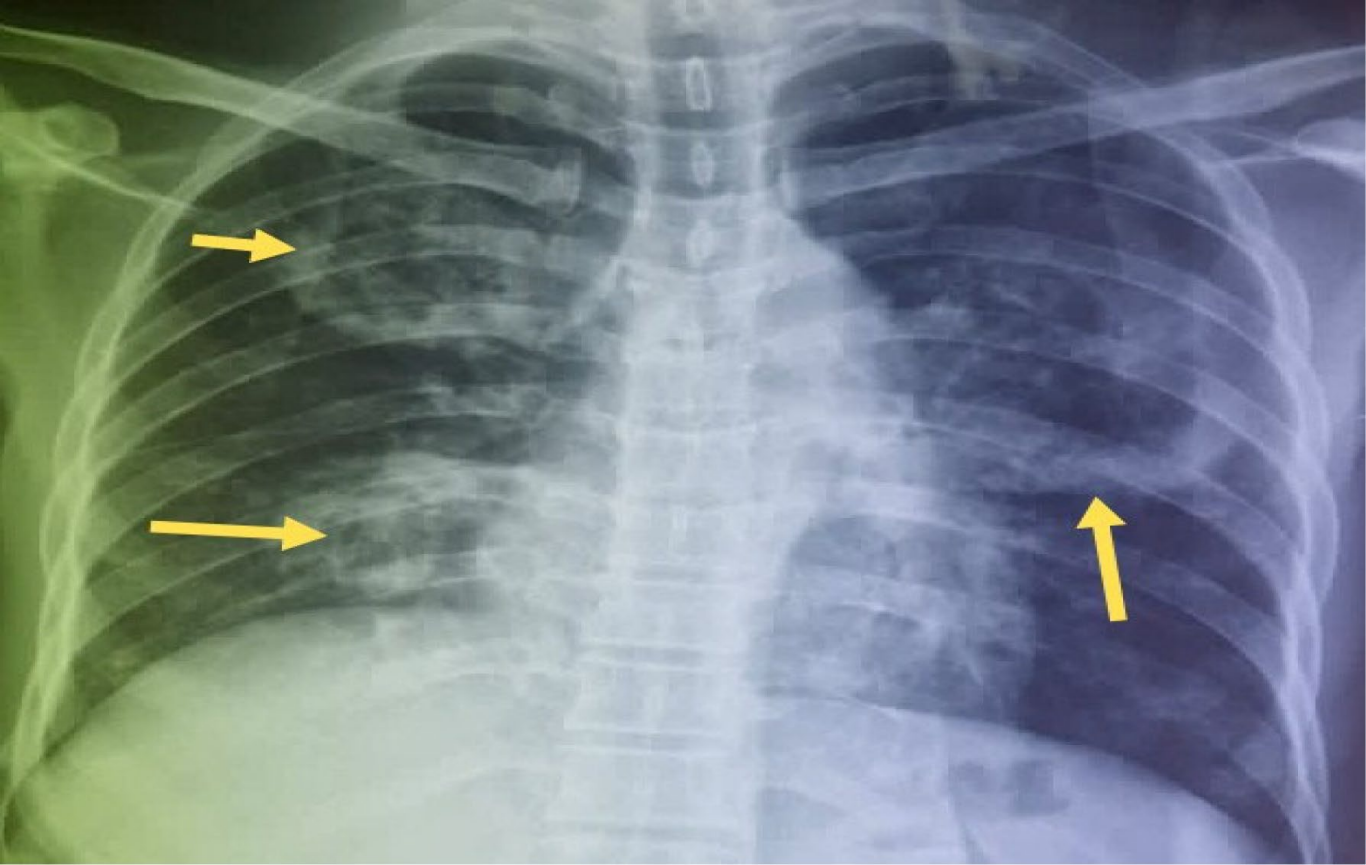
Posteroanterior (PA) chest radiograph showing cavitary lesions (yellow arrow) in the right and left lungs.
